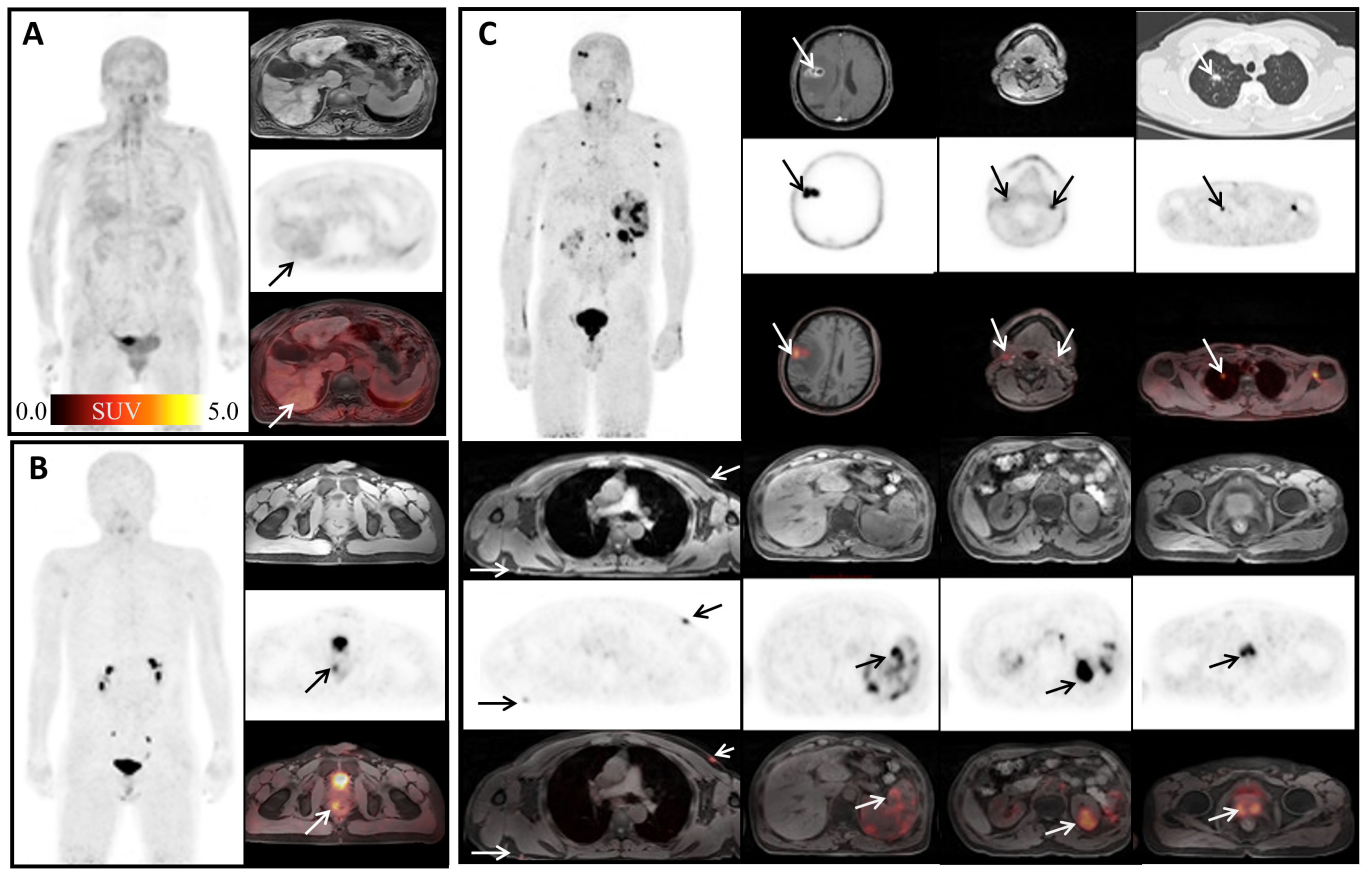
Interesting cases ^68^Ga-FAPI-04 imaging. (A) A 65-year-old woman with a history of hepatitis B over 30 years, arrows, cirrhosis of the liver, SUV_max_ 3.24; (B) a 19-year-old man with a 2-year history of rectal Crohn’s disease, arrows, rectal Crohn’s disease, SUV_max_ 5.22; (C) a 56-year-old man diagnosed with disease of disseminated non-tuberculous mycobacteriosis. The maximum intensity projection (MIP) image shows various FAPI-avid nodules: brain (SUV_max_ =2.61), cervical lymph nodes (SUV_max_ =1.81), upper lobe of right lung (SUV_max_ = 1.74), subcutaneous nodule on the left chest (SUV_max_ = 3.52), spleen (SUV_max_= 2.03), left kidney (SUV_max_ = 2.76), and prostate (SUV_max_ = 4.02).
